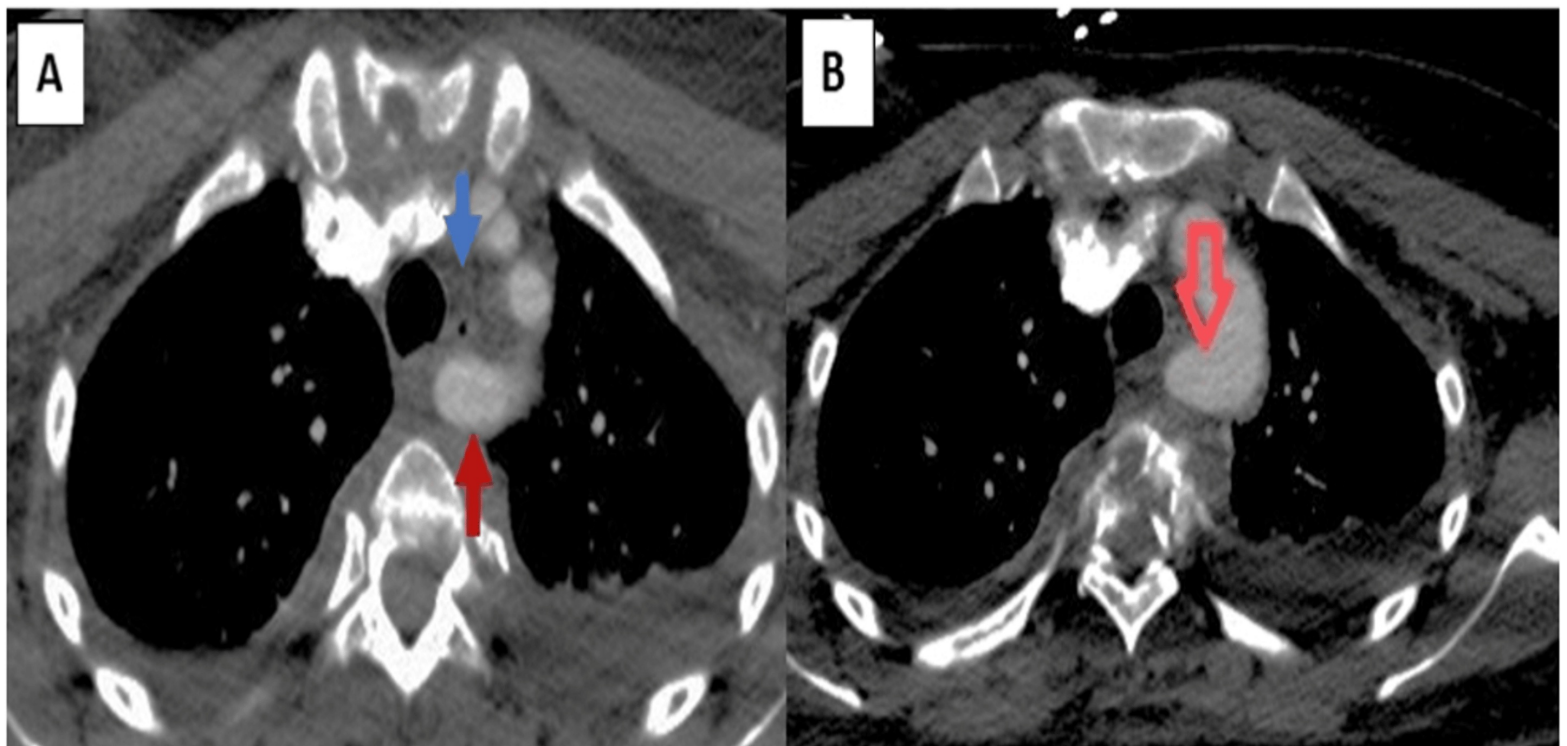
Axial (A and B) CT angiography A right subclavian artery (lusoria) (solid red arrow) arising from a Kommerell diverticulum (red arrow) at the level of the bone fragment compressing the aortic arch with no signs of esophageal or tracheal compression (blue arrow). The left subclavian artery originates from the anterolateral side of the aortic arch in front of the origin of the right subclavian artery. The common carotid arteries on the right and left are unremarkable.
